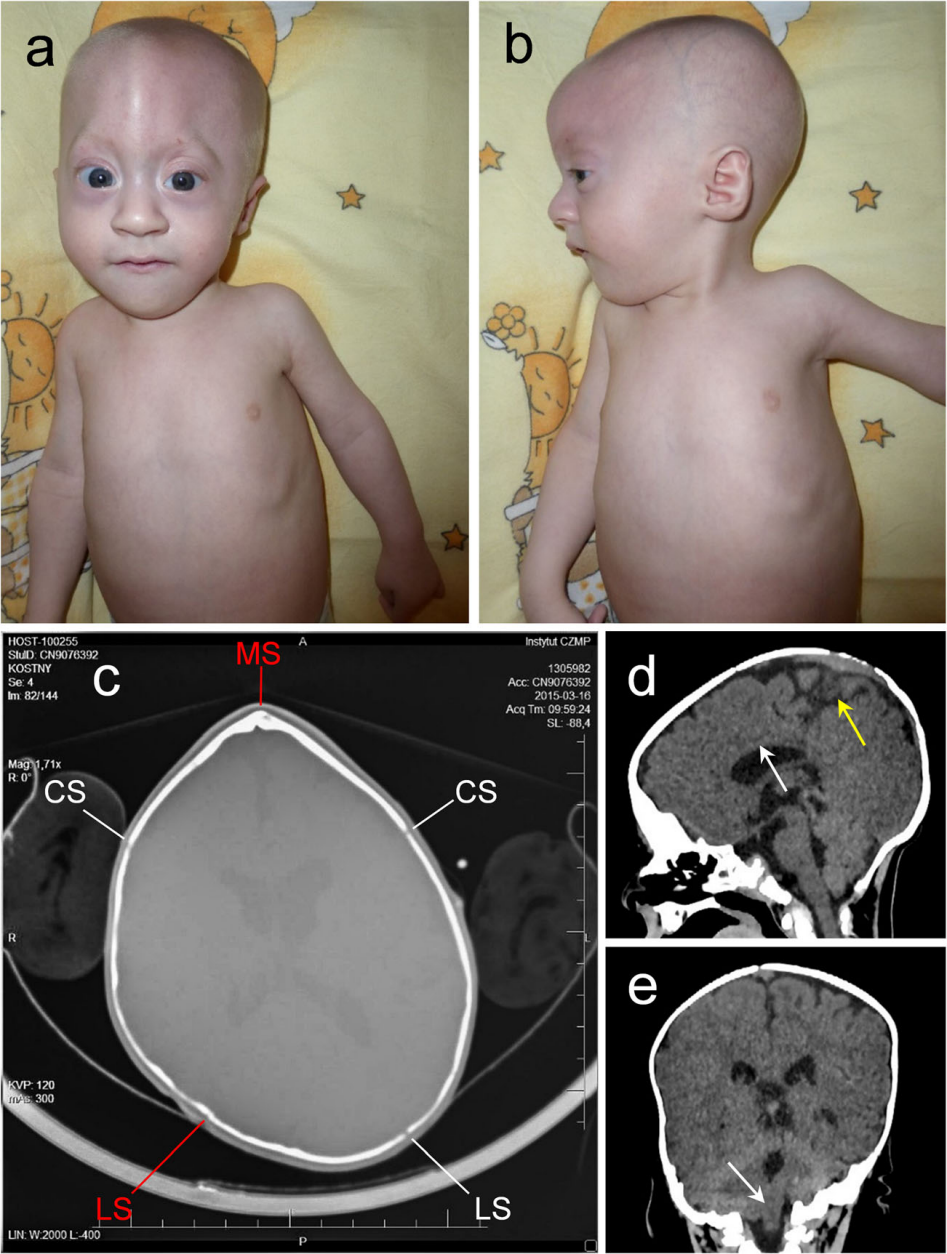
Facial view of the proband showing craniofacial abnormalities comprising a trigonocephaly with prominent metopic ridge, shallow orbits, proptosis, and hypotelorism and b flat facial profile, prominent occiput, and low-set, posteriorly rotated ears. c CT scan of the head showing premature synostosis of the metopic suture (trigonocephaly) and right lambdoid suture (indicated in red). MS—metopic suture, CS—coronal suture, LS—lambdoid suture. d CT scan of the head (sagittal view) showing thin and hypoplastic corpus callosum (marked with a white arrow) as well as morphological abnormalities of the median brain structures, including abnormal development of the white matter (marked with a yellow arrow). e CT scan of the head (coronal section) showing Chiari malformation; white arrow indicates downward displacement of the right cerebellar tonsil through the foramen magnum
